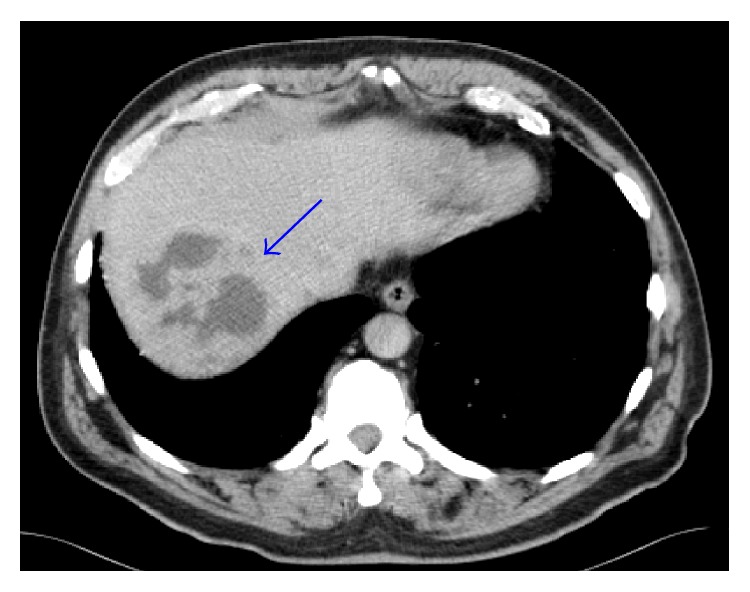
Loculated liver mass, as soon on admission CT.
